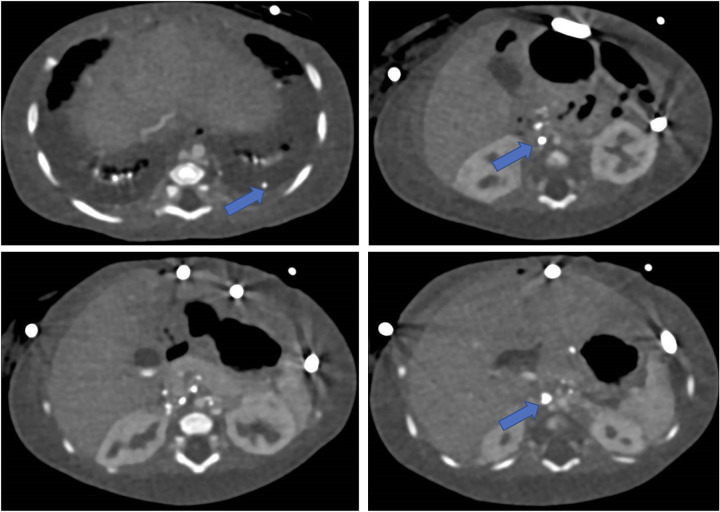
CT imaging demonstrating pleural and abdominal calcification nodules.
